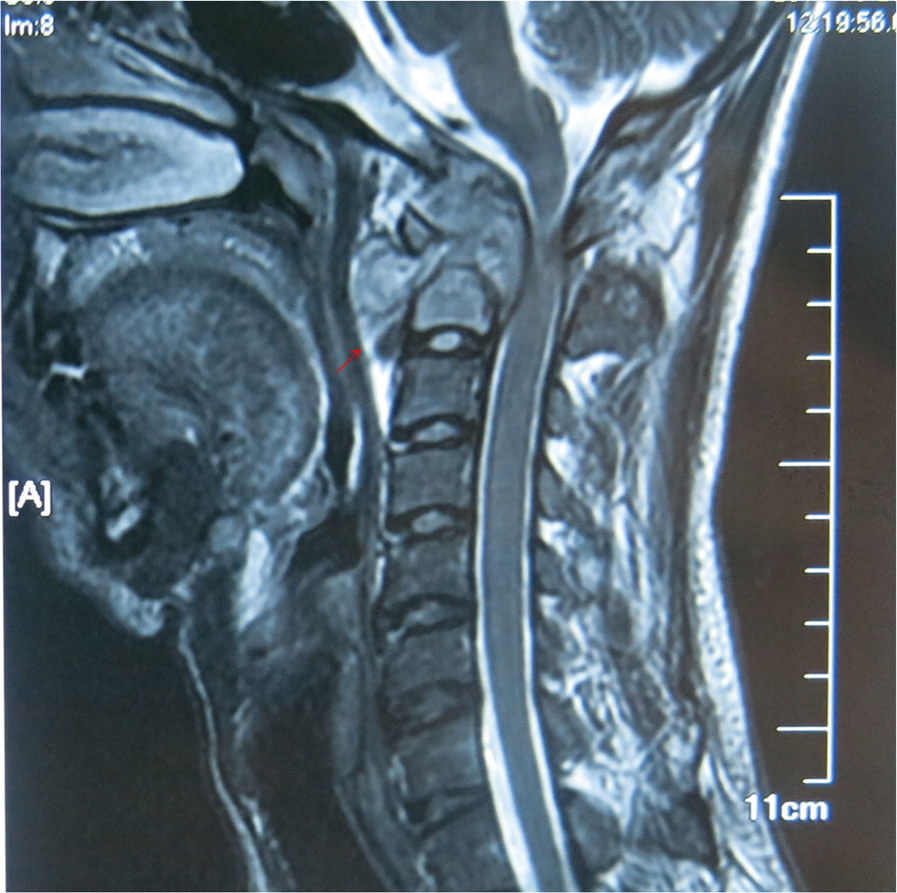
Preoperative MRI examination showed formation of atlantoaxial paravertebral abscess and retropharyngeal absess. The tuberculosis lesions spread into the spinal canal, leading to obvious compression of the spinal cord at C1–2 segments
